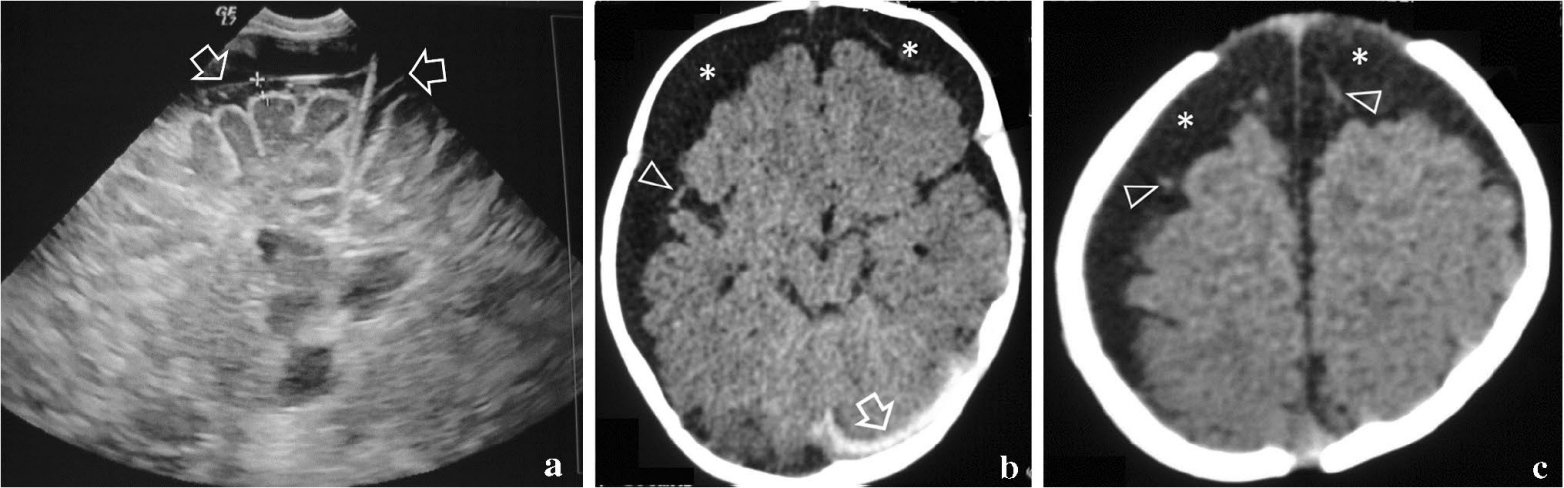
A 3.5-month-old abused boy was shaken and thrown against the floor in public by his alcoholic father. He was brought to hospital by the police with irritability, unalert gaze, bulging anterior fontanelle, generalised hypertonia, and a head circumference above the 97th percentile. a Coronal ultrasound, showing visible arachnoid membranes bilaterally (*arrows*), clearly separating the pericerebral spaces into subdural and subarachnoid (*between cursors*) compartments. b, c Axial computed tomography scan images demonstrate convexity subdural collections with cerebrospinal fluid density (*), a hyperdense infratentorial subdural haematoma (*arrow*) and subarachnoid vessels displaced against the brain’s surface (*arrowheads*). Such imaging findings should always rise concerns for trauma and should not be misinterpreted for benign enlargement of subarachnoid spaces
